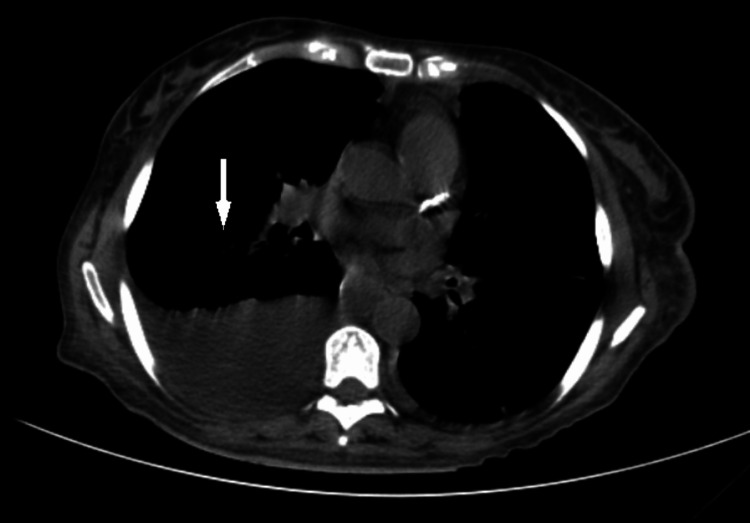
Lung CT showing bilateral pleural effusion
